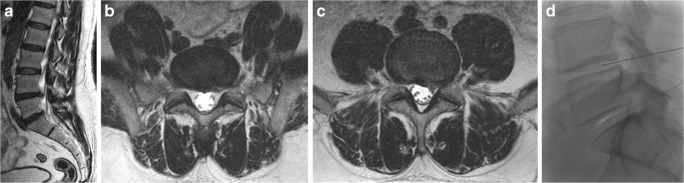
A 57-year-old man complaining of left lumbosciatica of 10-month duration and treated by O_2_-O_3_ chemonucleolysis. a Sagittal MRI T2-weighted image shows diffuse degenerative disc changes with small disc protrusions at the lower two lumbar levels. b, c The corresponding axial MRI T2-weighted images show left postero-lateral protrusions; at the levels of L4–5 and L5-S1, more evident at the latter one. d Lateral fluoroscopic image of the same patient shows the needles inside the center of corresponding discs, with ozone gas displayed as white linear opacity at L5–1 disc space
